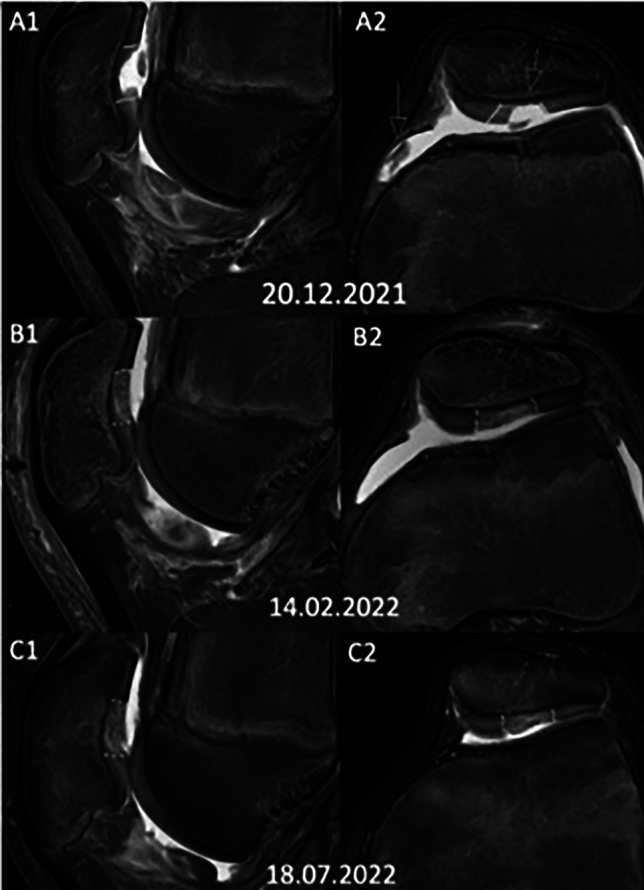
A1/A2 MRI scan of traumatic full-thickness cartilage defect at the central patella (arrows, defect zone yellow lines). B1/B2 MRI scan 6 weeks after surgery showing comminuted cartilage and synovial sealant. C1/2 MRI scan 6 months after the procedure. After complete rehabilitation, the patient is pain free and has already resumed his ice hockey career
